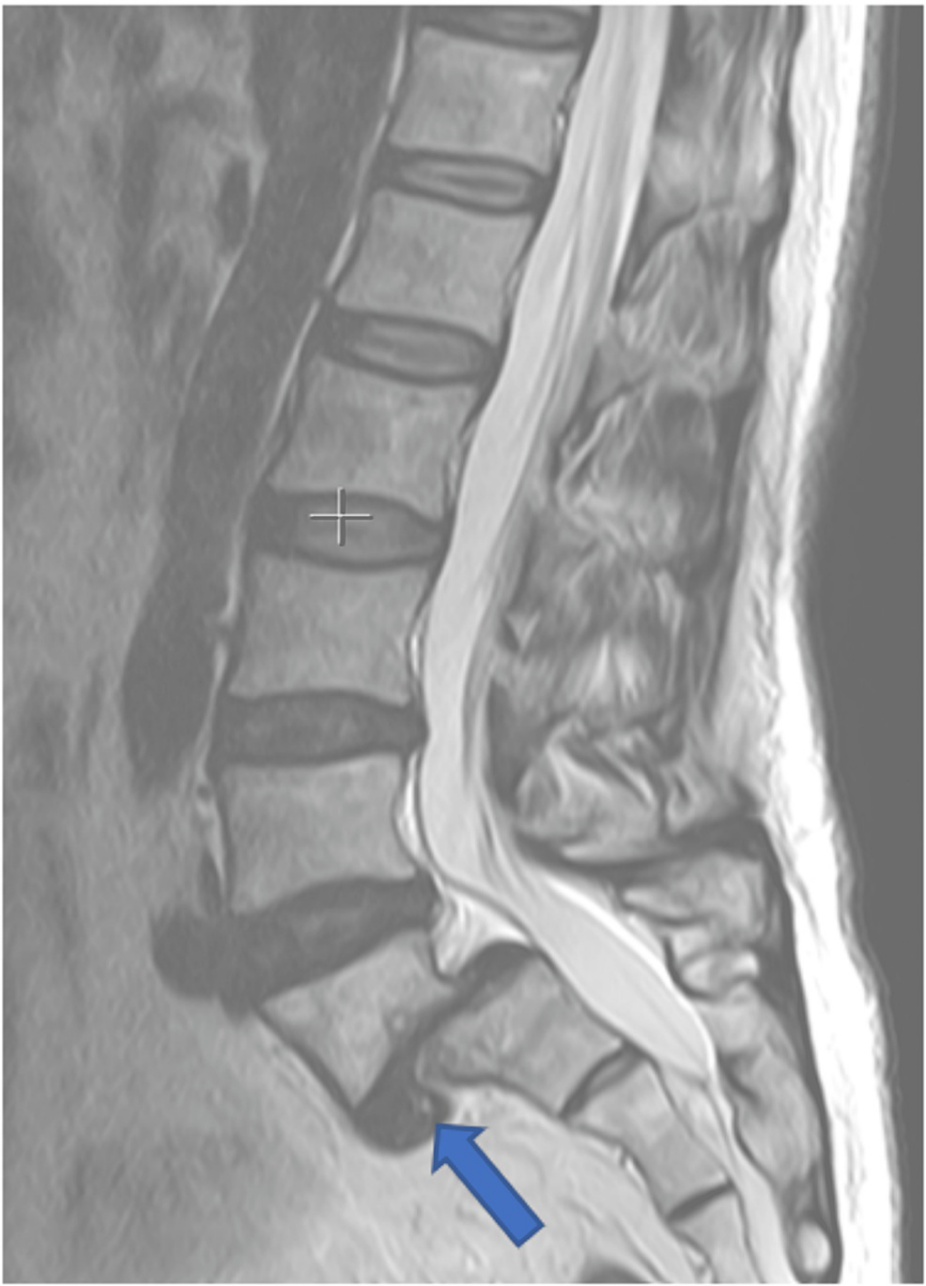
Preoperative sagittal MRI. It shows the L5 anterolisthesis with complete disc collapse and anterior disc herniation (blue arrow) with no modic changes. There was no stenosis of the spinal canal.
